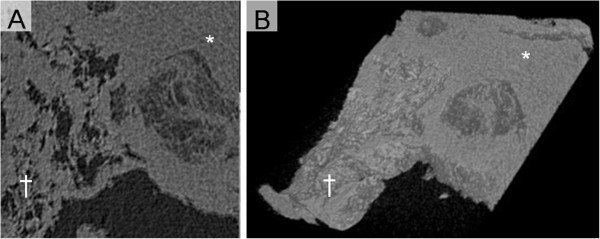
Radiological examinations in patient with invasive pulmonary mucormycosis presenting reversed halo sigh. (A) Micro computed tomography (CT) image of the lung tissue with invasive pulmonary mucormycosis. The dagger (†) and the asterisk signs (*) are placed on the areas of ground-glass opacity (GGO) and outer rim, respectively. (B) 3D reconstruction image processed by applying 3D volume rendering software. Central GGO and peripheral ring-shaped high-density area (*) were observed.
